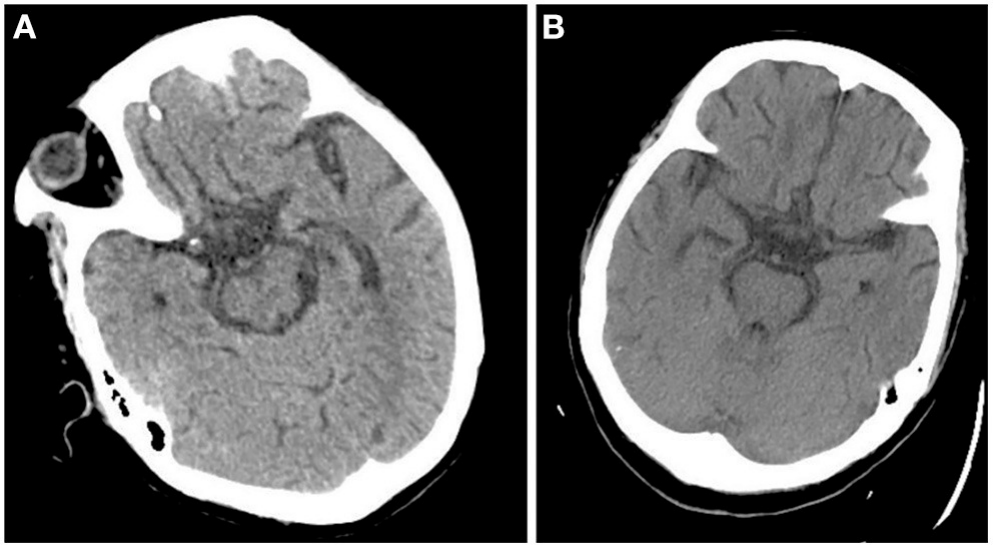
CT scan didn't demonstrate any hypodensity in pons 1 day after the operation (A) and 7 days after the operation (B).
